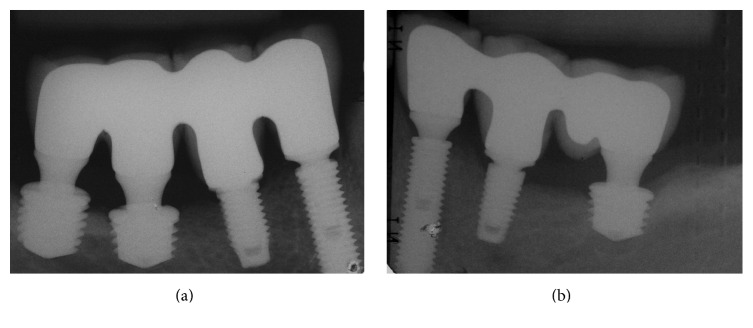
Periapical radiographs at the prosthesis installation session in the mandibular arch: (a) right and (b) left side.
